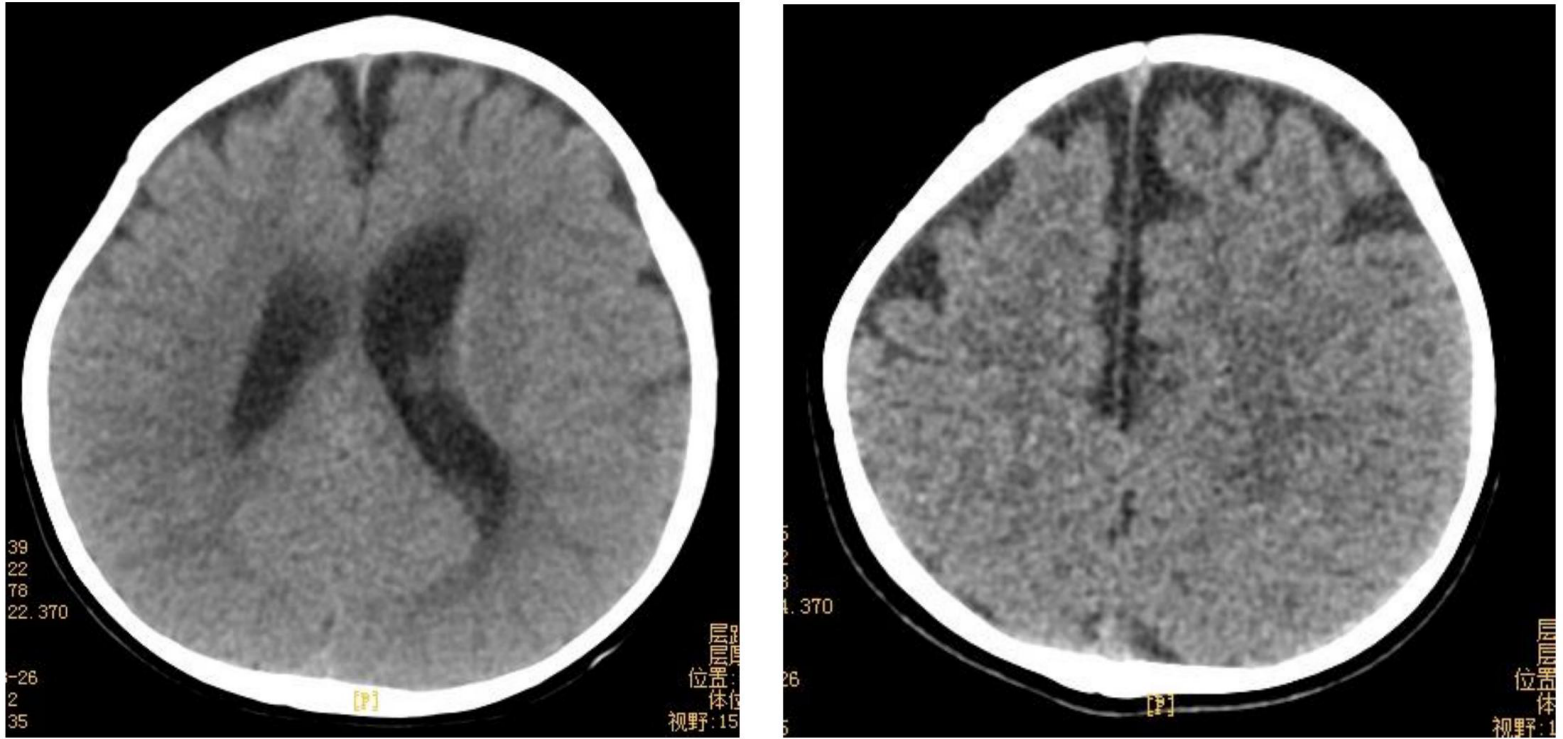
Cranial CT showed abnormal skull development and bilateral ventriculomegaly. Bilateral frontotemporal extracerebral Spaces were widened.
